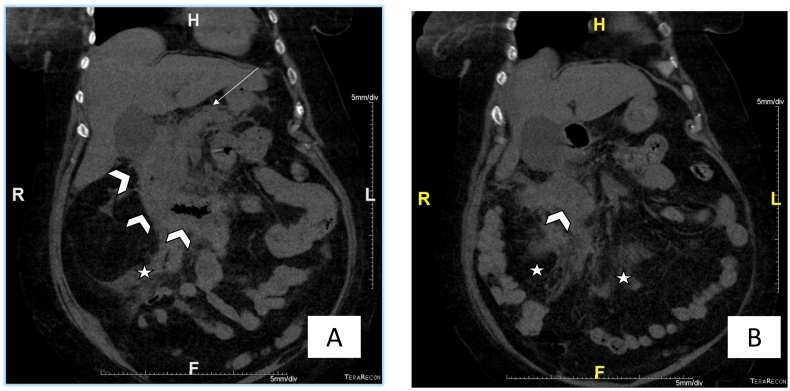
(A,B): CT coronal reconstructions without contrast injection showing a regular circumferential parietal thickening spontaneously hyperdense of the D2, D3 portions of the duodenum (arrowheads), swollen pancreas (arrow) with densification of the mesenteric fat around (star).
